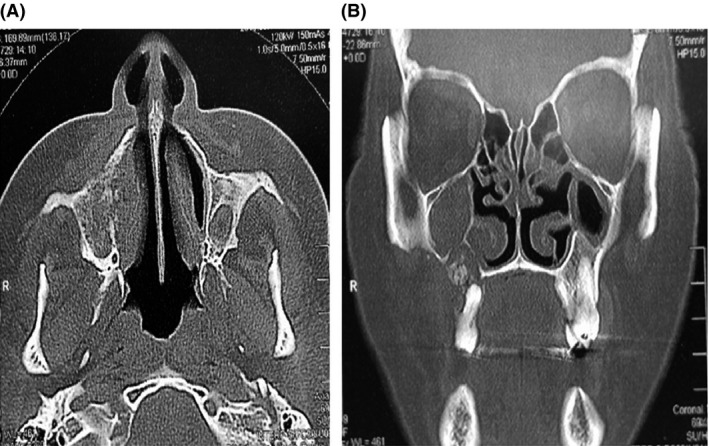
Computed tomography of the lesion before the first biopsy. Bone necrosis and right oroantral communication are present. (A) axial cut. (B) coronal court.
